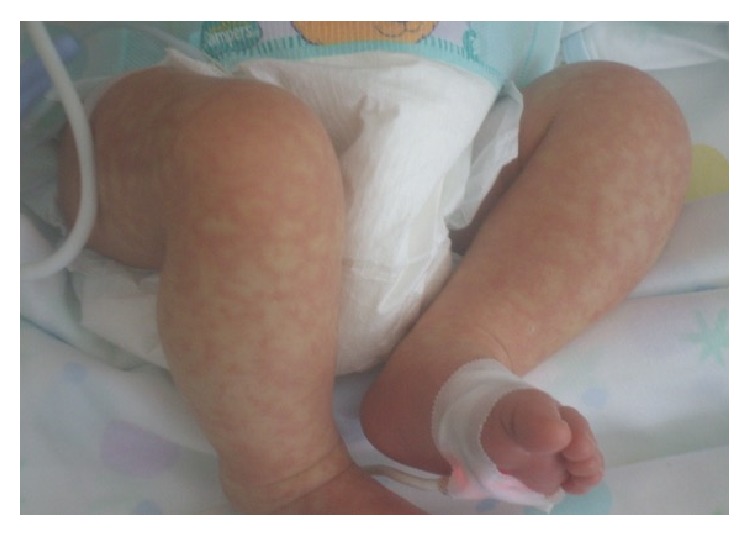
A reticulated, maculopapular rash in the lower limb in a term male affected by haemophagocytic lymphohistiocytosis. He presented with fever associated with generalized rash which disappeared in the first week of life and appeared again on 30th day. Blood test analysis showed values indicative of liver failure; blood cell count revealed low platelets values. The abdominal ultrasound scan showed thinly irregular liver parenchyma. Hypoplasia of granulocyte and erythroblastic and megakaryocytic lineages (<5% each) with haematopoietic cell phagocytosis by mature macrophages were present in the bone marrow aspirate. High-dose glucocorticoids, cyclosporine, and etoposide were started. Despite the therapy, there has been gradual deterioration of the clinical condition until coma, respiratory failure, intractable hypotension, and coagulopathy led to death despite intensive vital support.
